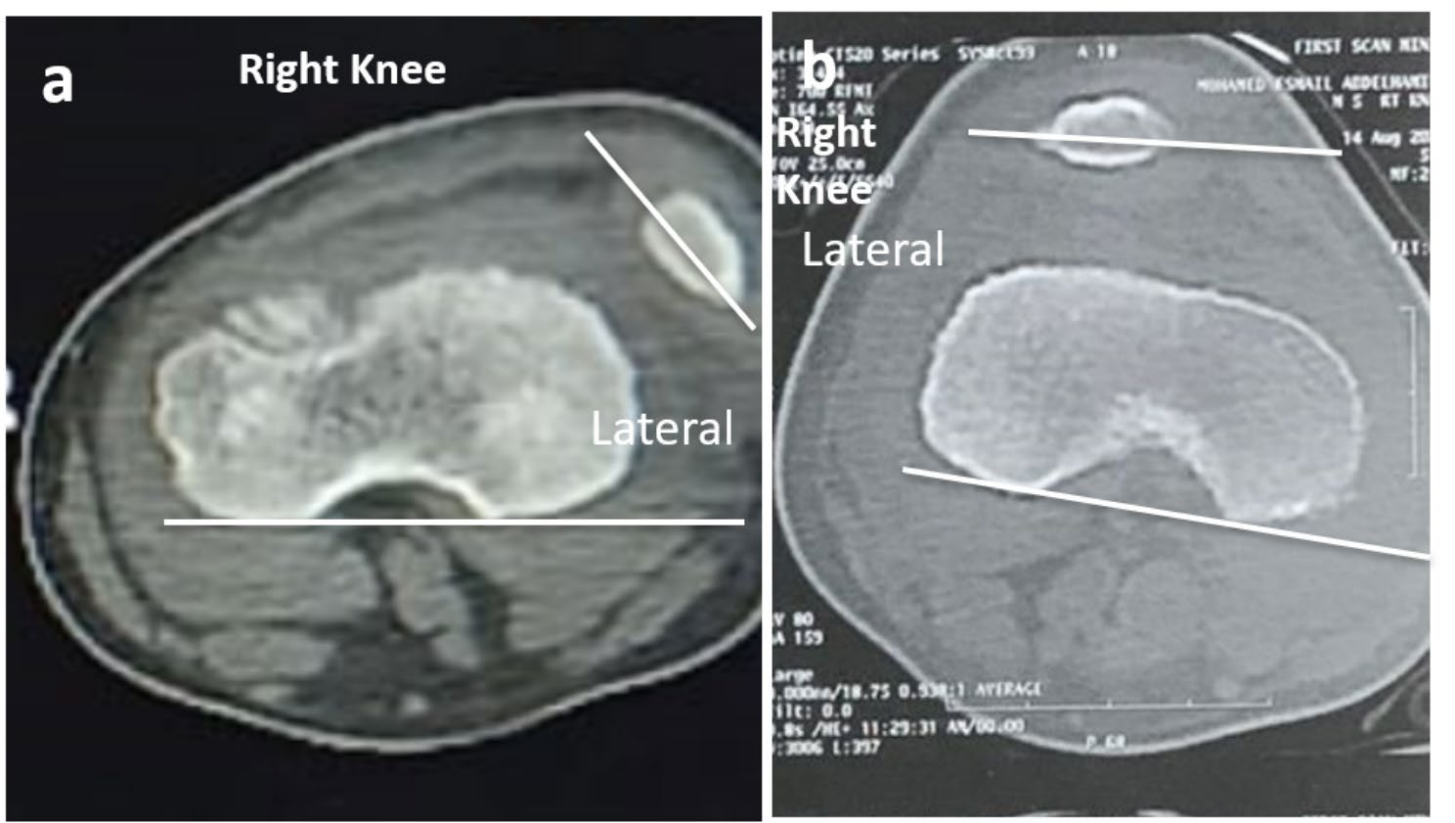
Computed Tomography CT scans. (a) Preoperative axial CT scan of the right knee showing lateral patellar subluxation and the patellar tilt angle (PTA; the angle formed between a line defining the maximal width of the patella and the femoral posterior condylar line; 44.6^0^). (b) Post-operative final axial CT scan of the same knee showing an improved PTA (1.8^0^) and a centralized patella
